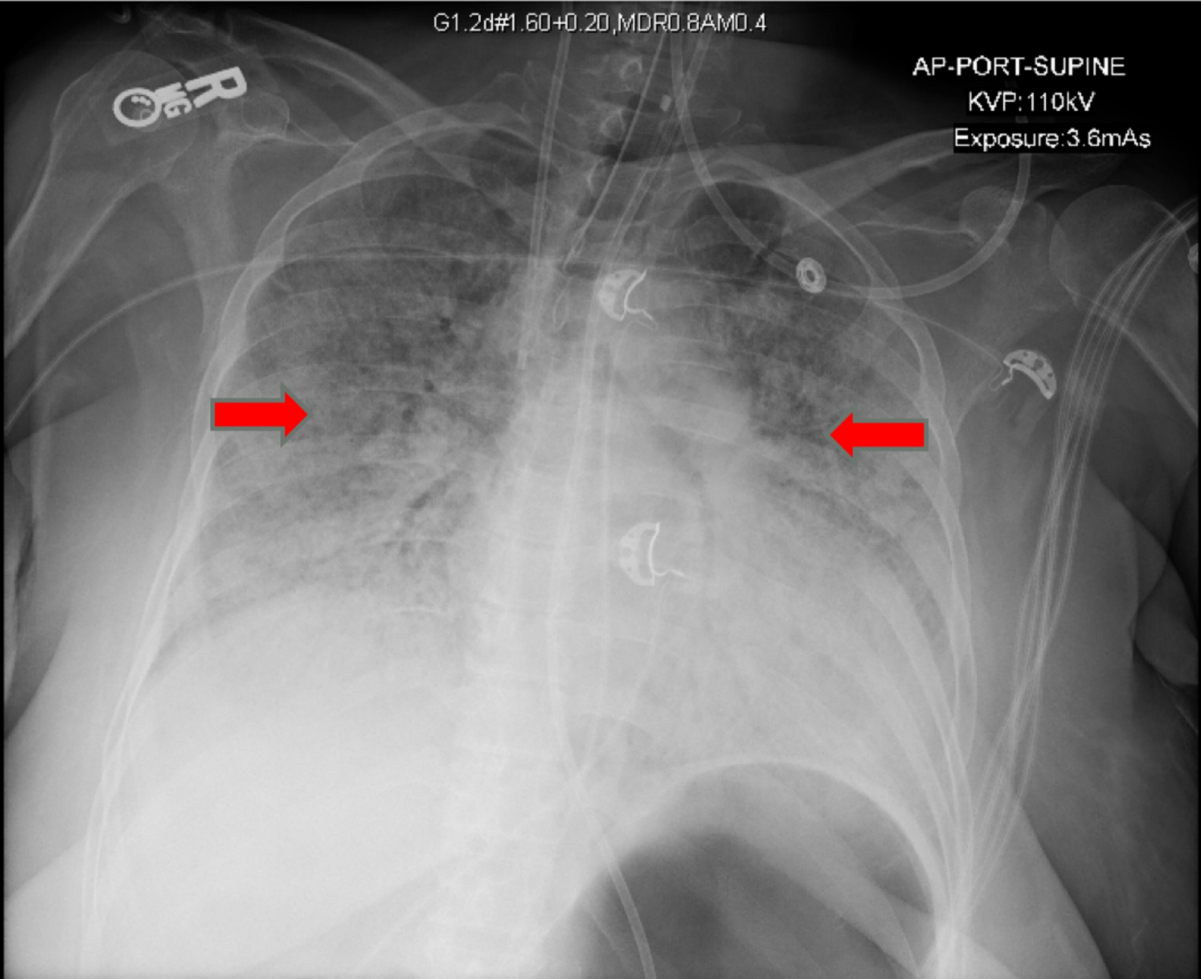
X-ray after intubation Endotracheal tube projects at the upper margin of the right clavicle, rotated, right internal jugular (IJ) temporary hemodialysis catheter in the right brachycephalic vein, nasogastric tube in place. Extensive diffuse pulmonary confluent airspace opacities.
